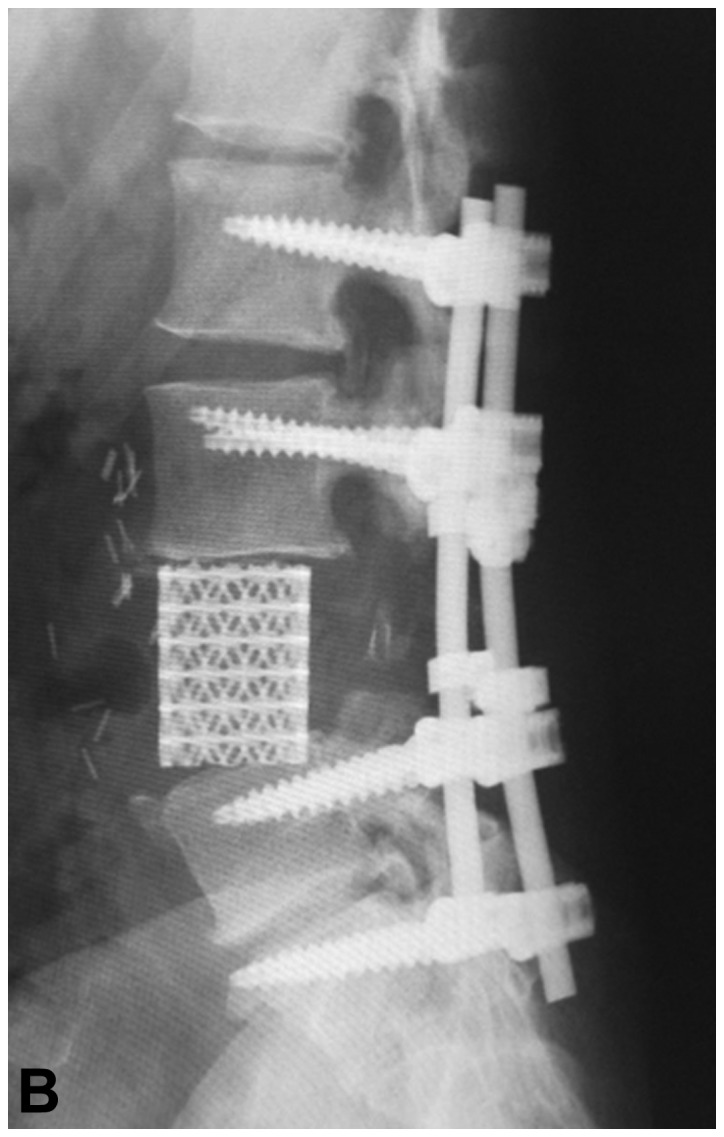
Radiographic findings following the second surgery. (A) Anterior-posterior and (B) lateral views.
